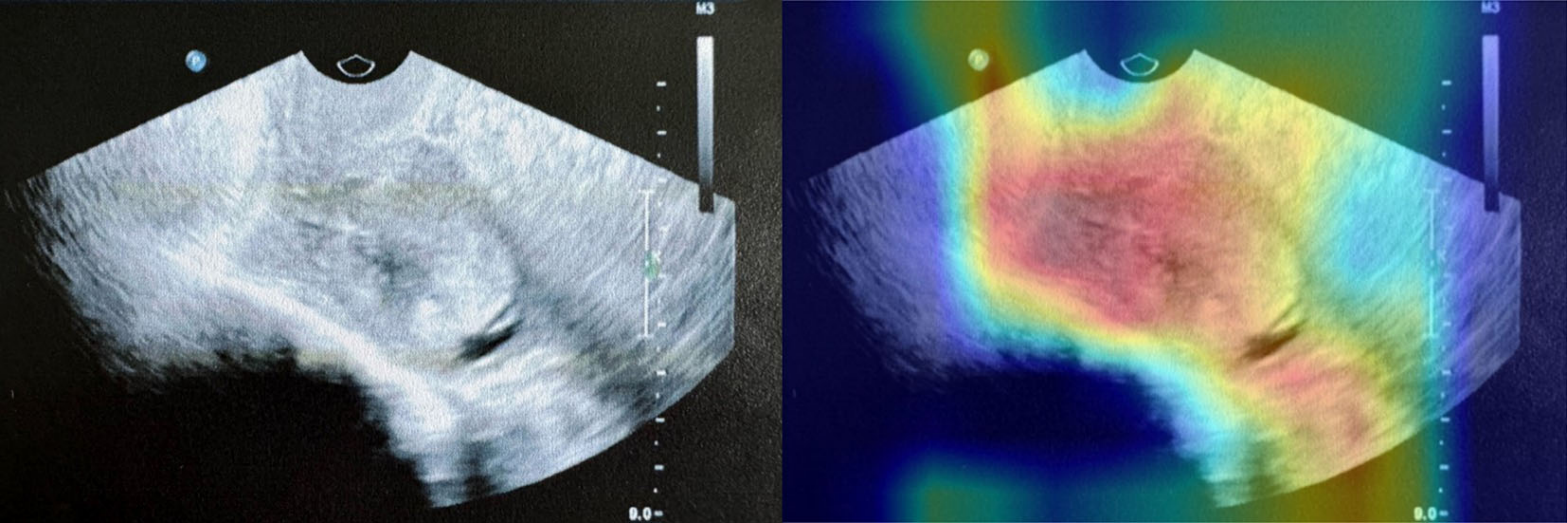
An example of Grad-CAM interpretability analysis in testing set. The left panel shows the original ultrasound image, while the right panel overlays the Grad-CAM heatmap, highlighting the regions to which the model paid the most attention during prediction.
